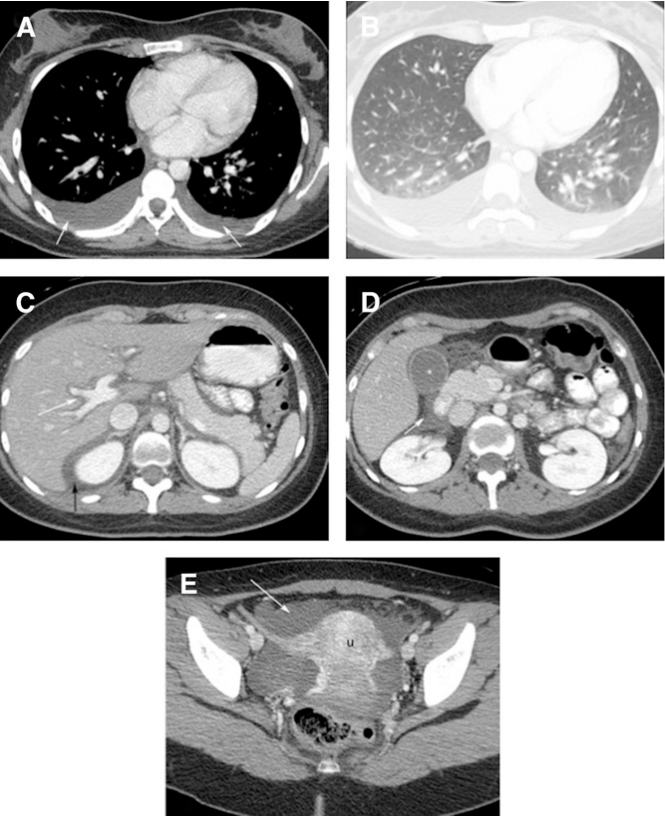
Computed tomography (CT) of the abdomen including the lung bases (A) showed bilateral pleural effusions without evidence of pulmonary edema on lung windows (B). Moderate amount of free fluid seen in the upper abdomen (C, D) with periportal edema (white arrow; C), and fluid in Morrison's pouch (black arrow; C). Pericholecystic fluid (white arrow; D) surrounding the gallbladder (* D) is shown. Intraperitoneal fluid is also seen in the pelvis (white arrow; E) outlining the uterus (u).
